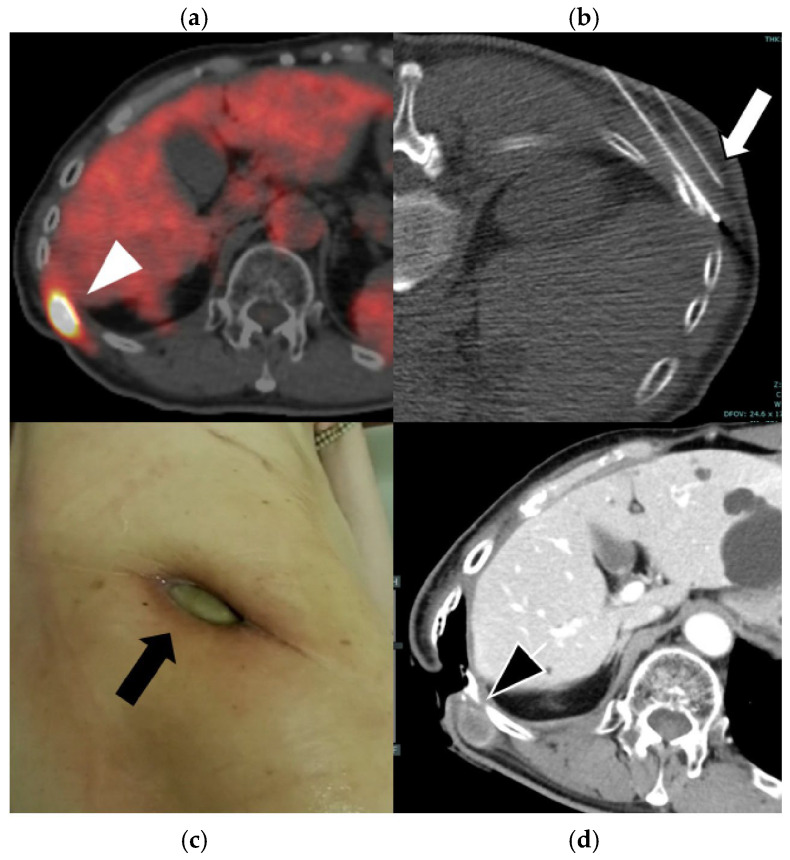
Images of a 60-year-old man with epithelioid pleural mesothelioma. Baseline axial 18F-FDG PET/CT image (a) showing focal avid FDG uptake in the right chest wall (white arrowhead). Despite undergoing multiple treatments, including surgery, radiation therapy, and systemic therapy, the recurrent tumor remained uncontrolled, and RFA was performed. The procedure was conducted with hydrodissection in the subcutaneous tissue (white arrow) and concurrent skin cooling using an ice pack to prevent thermal injury (b). However, a skin burn developed the following day, progressing to a refractory skin ulcer (black arrow) (c). Follow-up CT revealed local tumor progression (black arrowhead), but the patient declined further treatment (d).
